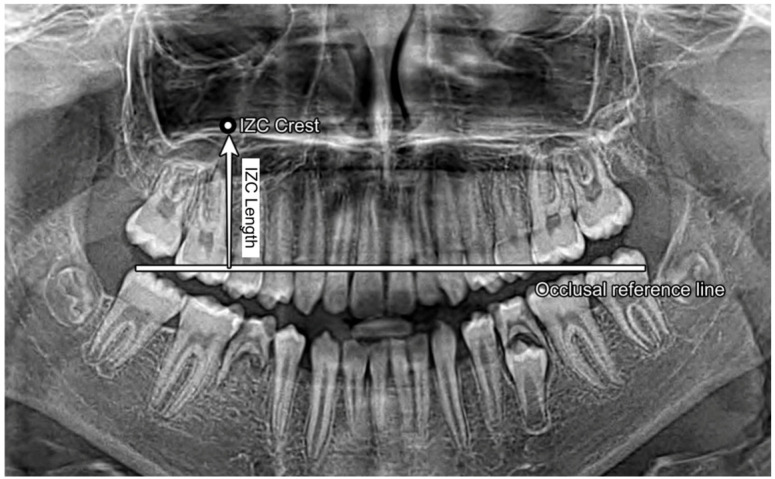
Measurement of panoramic infrazygomatic crest (IZC) length on panoramic radiographs. The occlusal reference line connects the mesiobuccal cusp tips of the maxillary first molars. Panoramic IZC length was defined as the perpendicular two-dimensional radiographic distance from this reference line to the most concave point of the zygomatic buttress contour on the panoramic image. This measurement was used for radiographic morphometric comparison and does not represent three-dimensional bone depth, cortical thickness, sinus proximity, or the oblique extra-alveolar insertion path used for IZC miniscrews.
